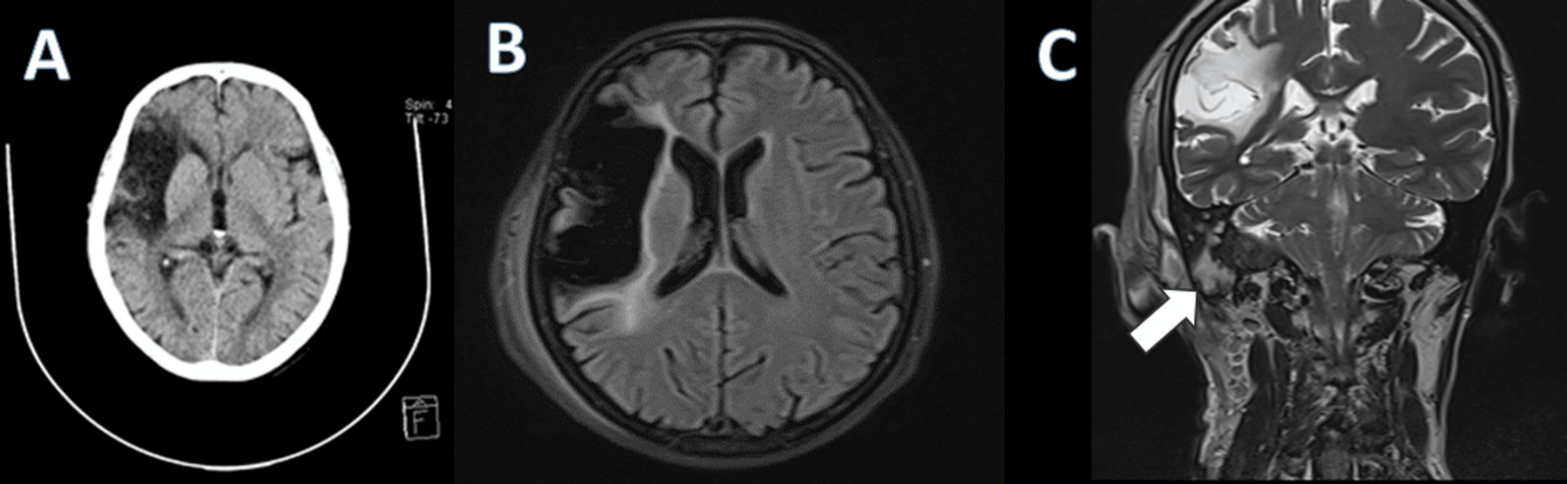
Presence of leukoencephalomalacia on computed tomography and MRI A. Axial CT: Chronic ischemic stroke; B. Axial FLAIR MRI: Chronic ischemic stroke and gliosis; C. Coronal T2 MRI: Jugulotympanic glomus (white arrow) FLAIR: fluid-attenuated inversion recovery
